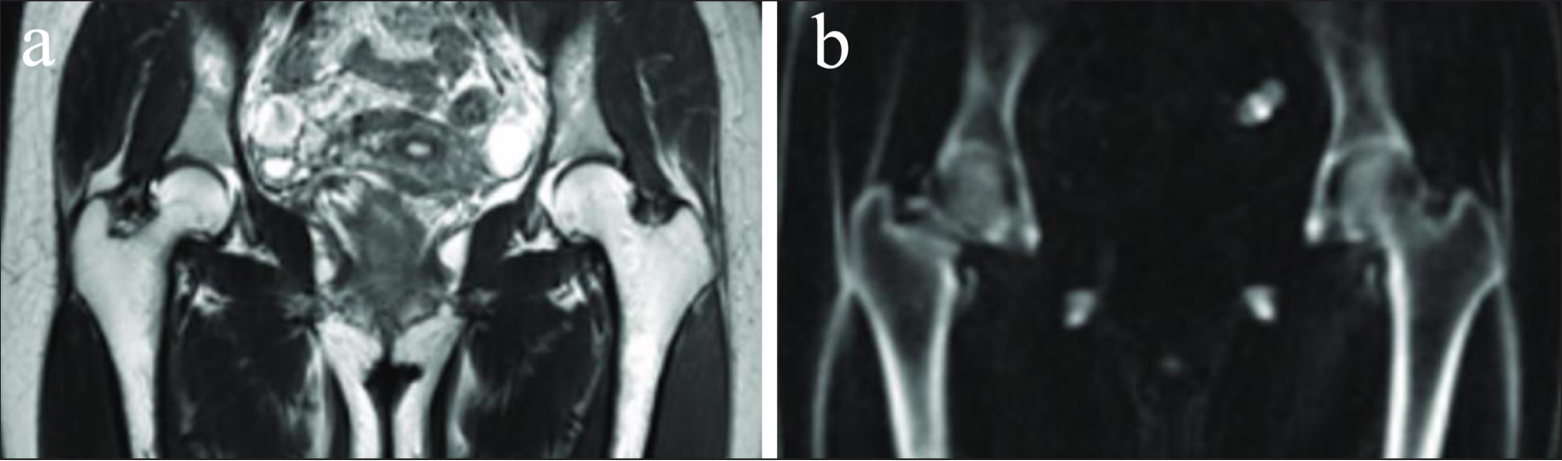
T2-weighted (T2W) image versus ultrashort echo time (UTE) image. In vivo imaging of the hip with a 2D T2W fast spin echo sequence (a) and 3D Inversion Recovery (IR) UTE Cones sequence (b). Soft tissues are well-suppressed in the 3D IR-UTE Cones image, while they are bright in the T2W images. Figure reproduced from Jerban et al. [23] under the Creative Commons Attribution 4.0 International License (CC Creative Commons — Attribution 4.0 International — CC BY-NC 4.0, https://creativecommons.org/licenses/by-nc/4.0/). Minor modifications were performed for presentation purposes.
